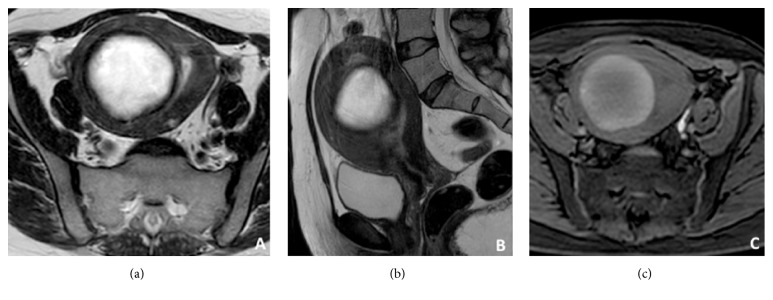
T2w MRI axial (a) and sagittal (b) images show an intramural myoma; T1W-FS MR axial image confirmed a well capsulated centrally hyalinized uterine lesion (c).
